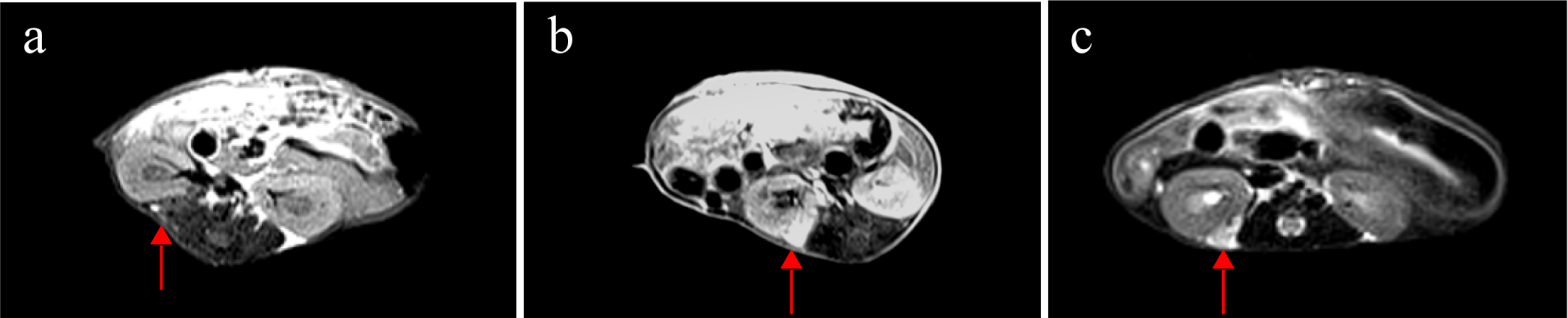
MRI findings of renal capsule PDX in T1WI, T1WI+C, and T2WI sequences(arrow). (a) Isointense and Hypointense on T1WI. (b) marked enhancement on T1WI contrast-enhanced(T1WI+C) scans. (c) Isointense and Hyperintense on T2WI, companying with renal compression. T1-weighted imaging (T1WI), T1-weighted contrast-enhanced imaging (T1WI+C), T2-weighted imaging (T2WI).
